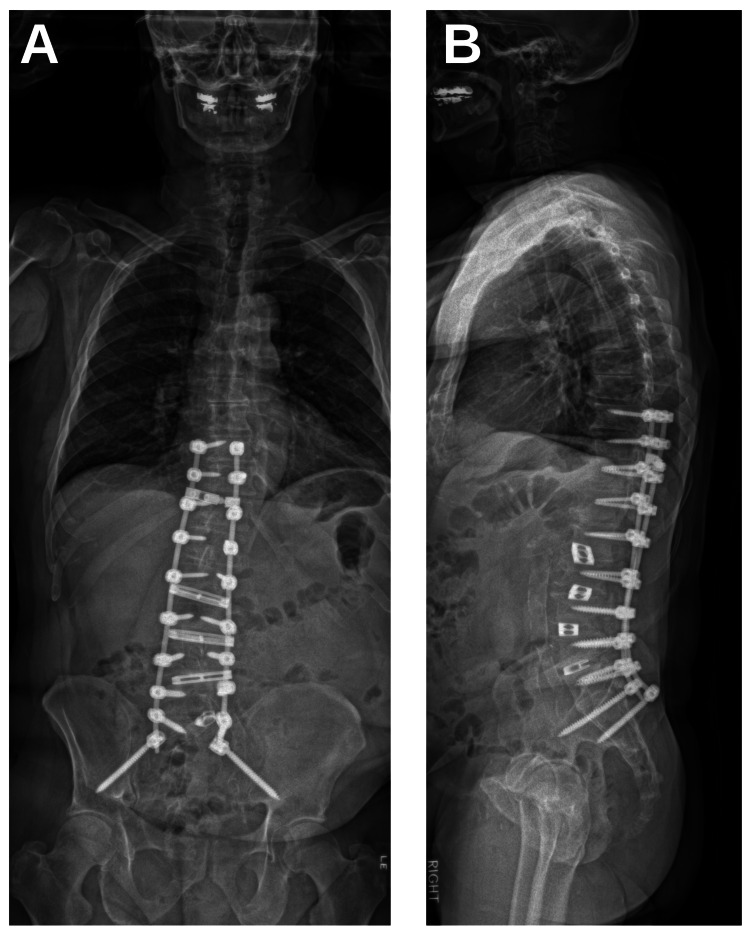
Post-Operative Radiography Post-operative coronal (A) and sagittal (B) radiography shows instrumentation and significant correction of scoliotic deformity.
